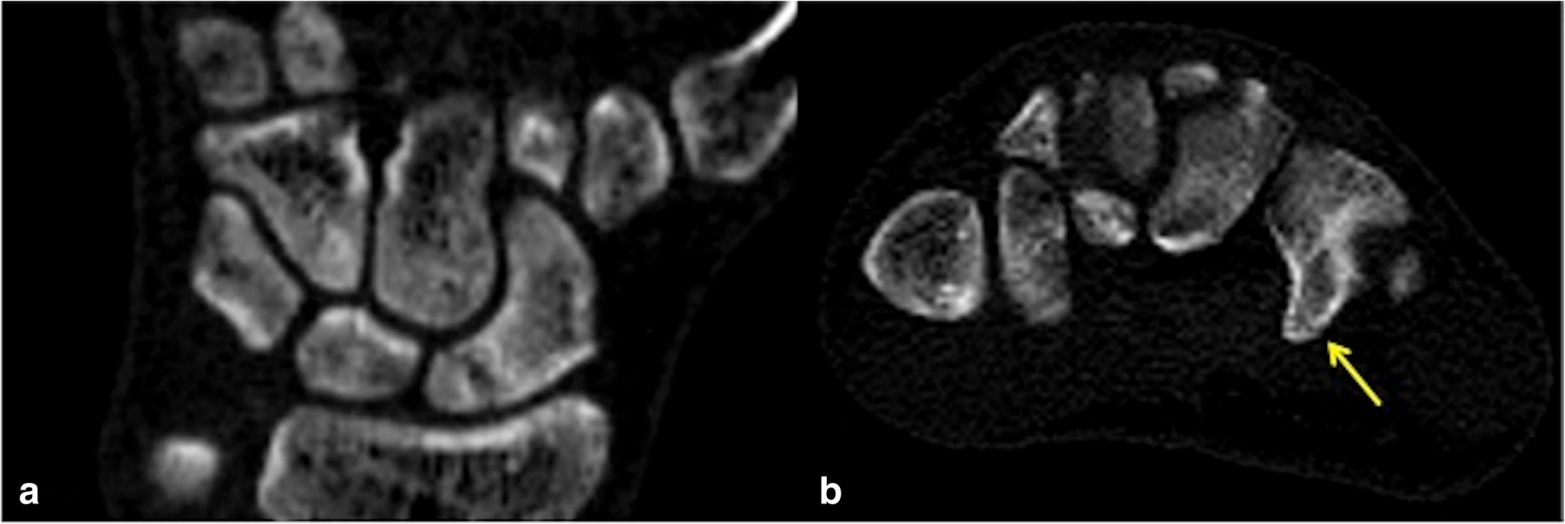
A 24-year-old man with fracture of the left scaphoid and concomitant hook of hamate fracture involving the tip of the hamate hook (CT performed 2 weeks after the initial injury). a Coronal CT reconstruction shows the minimally displaced fracture of the proximal pole of the scaphoid with some resorption of the fracture margins. b Axial CT image shows the subtle non-displaced concomitant fracture of the left hook of hamate tip (*arrow*), which is easily overlooked if not actively sought
